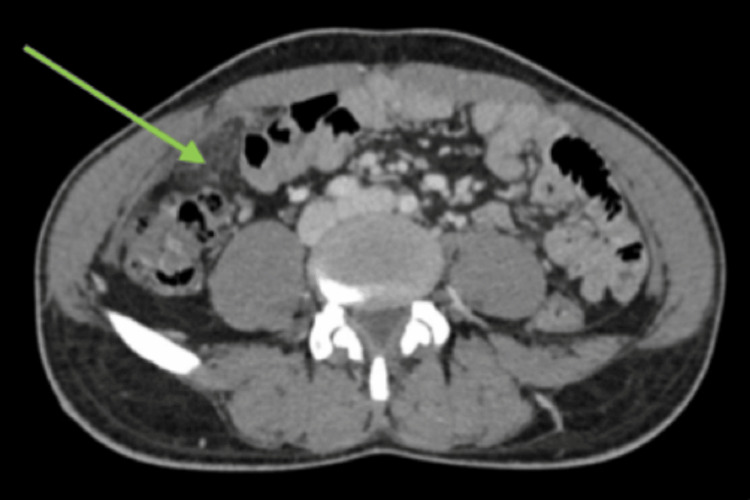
Axial contrast-enhanced CT of the abdomen demonstrating primary OI Axial contrast-enhanced CT image showing focal fat stranding and inflammatory changes in the right lower quadrant within the greater omentum (green arrow), consistent with OI. OI, omental infarction
